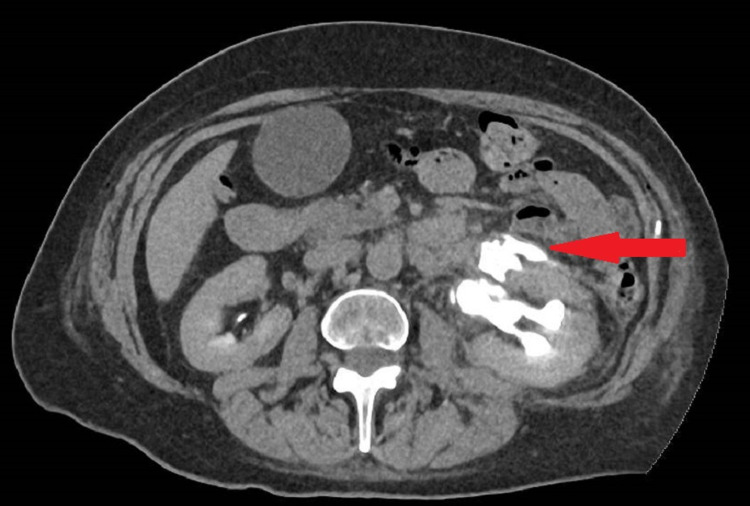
Axial contrast CT scan showing extravasation of the contrast at the left renal pelvis one week post traumatic injury (red arrow)
